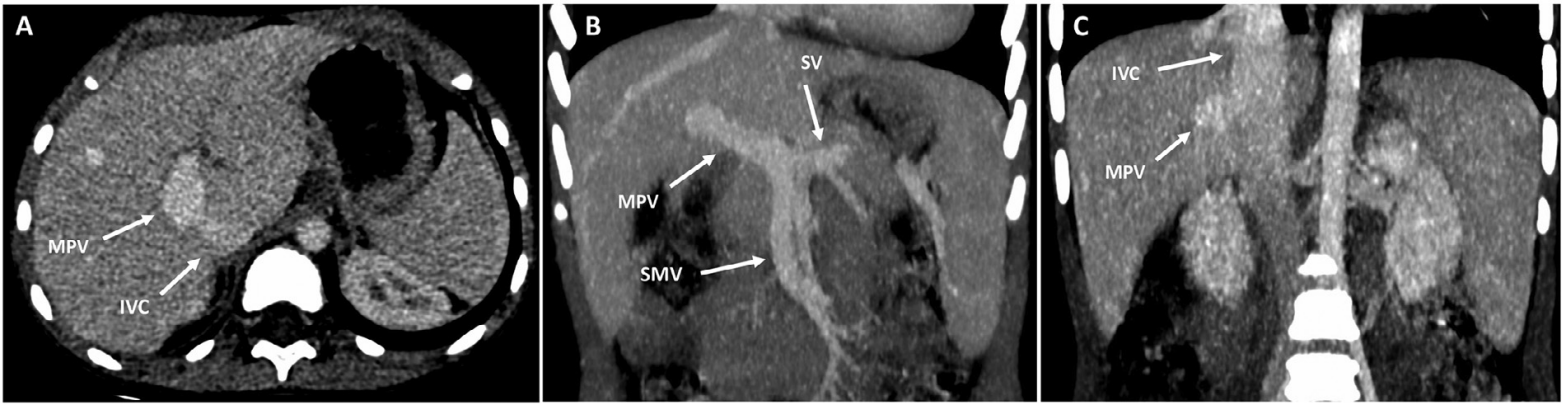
Abdominal CT in the portal venous phase. (A) CT in the axial plane shows a communication between the MPV and the IVC. (B and C) Maximum intensity projection (MIP) coronal reconstruction shows the MPV originating from the confluence of superior mesenteric vein (SMV) and splenic vein (SV) (B) and an anastomosis between the MPV and IVC (C). A homogeneous splenomegaly of 11 cm (long splenic axis) is also seen. CT, computed tomography; IVC, inferior vena cava; MPV, main portal vein.
